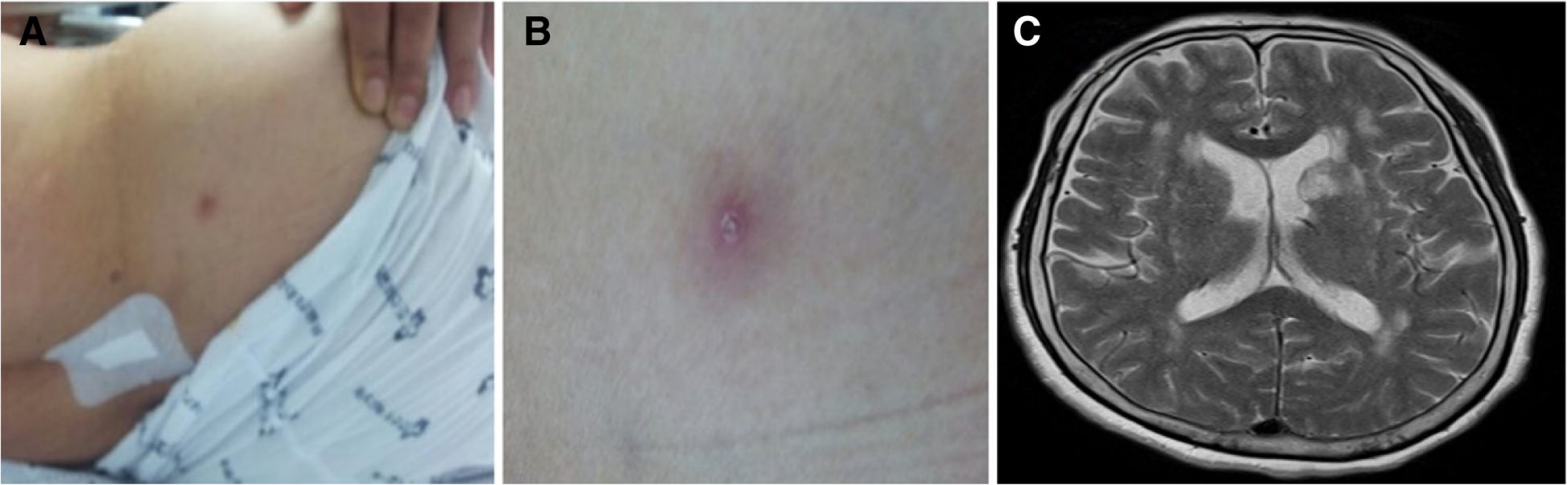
A 70-year-old female patient with a confirmed diagnosis of human granulocytic anaplasmosis. a A lesion suspected of being a tick bite found on the right buttocks area at the time of admission. b Magnified image of the site of suspected tick bite. c Right middle cerebral artery territory infarction (recent onset infarction)on T2 -weightedimage on brain magnetic resonance imaging
